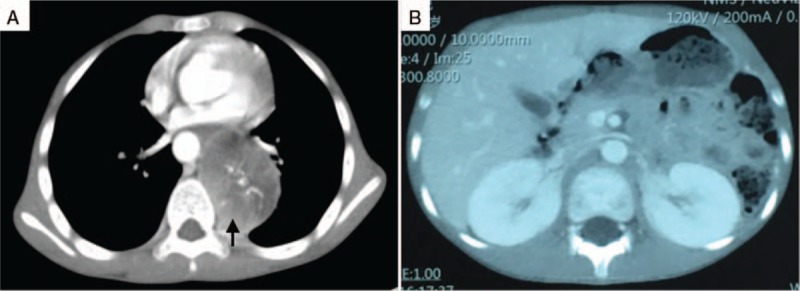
A, CT scan of the chest showed a 7 × 5-cm-sized soft tissue mass on the left paraspinal with destroying adjacent thoracic vertebra and ribs (arrow, the tumor). B, CT scan of the abdomen showed normal. CT = computed tomography.
